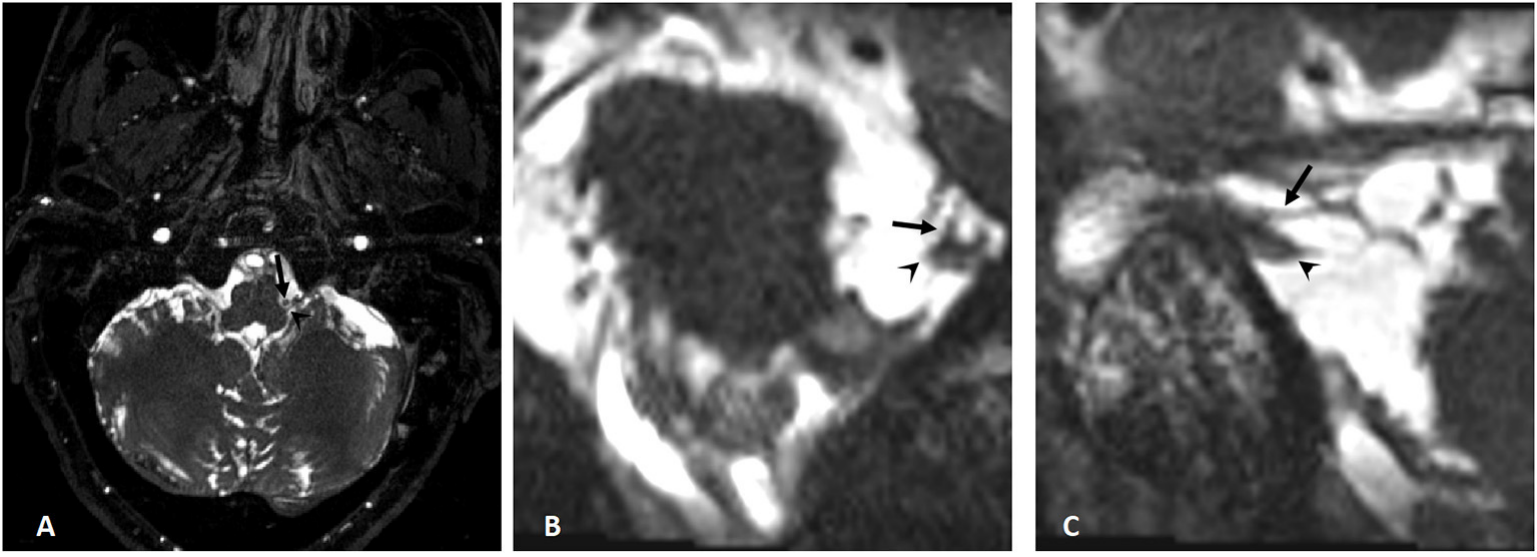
MRI before the second operation. (A) The structure around the left glossopharyngeal nerve is disordered, and the original posterior inferior cerebellar artery (arrow) seems to be moved with no compression to the left glossopharyngeal nerve (arrowhead); (B, C) The left trigeminal nerve (arrowhead) touches the superior cerebellar artery (arrow).
